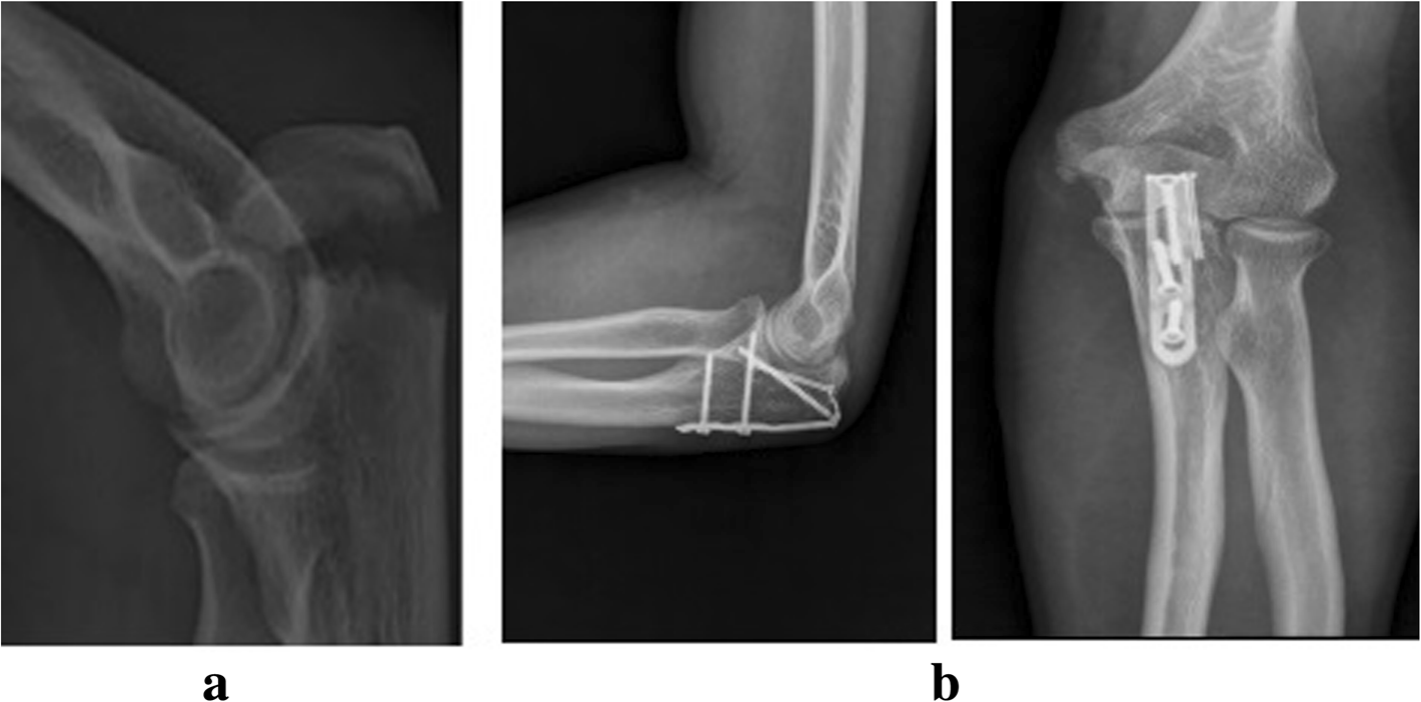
The radiographs of patients with left olecranon avulsion fracture treated with hook plate fixation. a Preoperative lateral X-ray films; b Posteroanterior and lateral X-ray films after 3 months postoperatively
